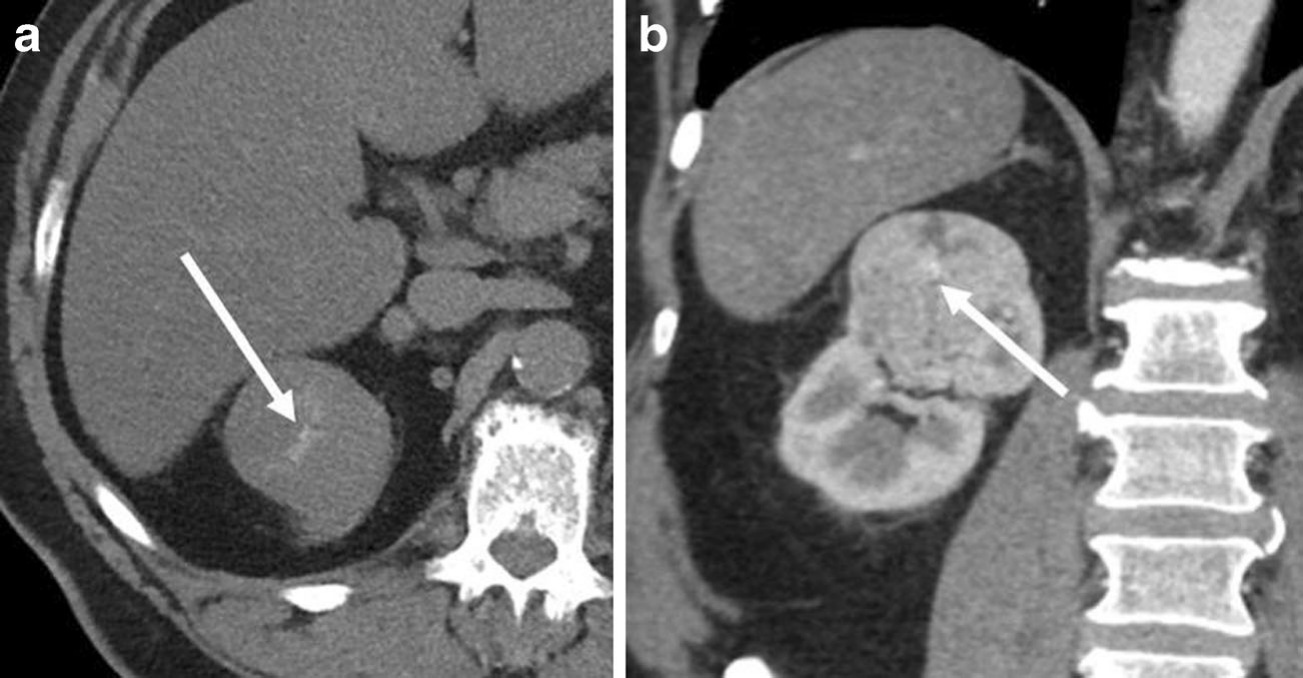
A 71-year-old man with a right renal oncocytoma with calcification. a The axial unenhanced CT demonstrates calcification (*arrow*) within the mass. b The coronal reformatted image in the corticomedullary phase shows calcification in the central scar (*arrow*)
